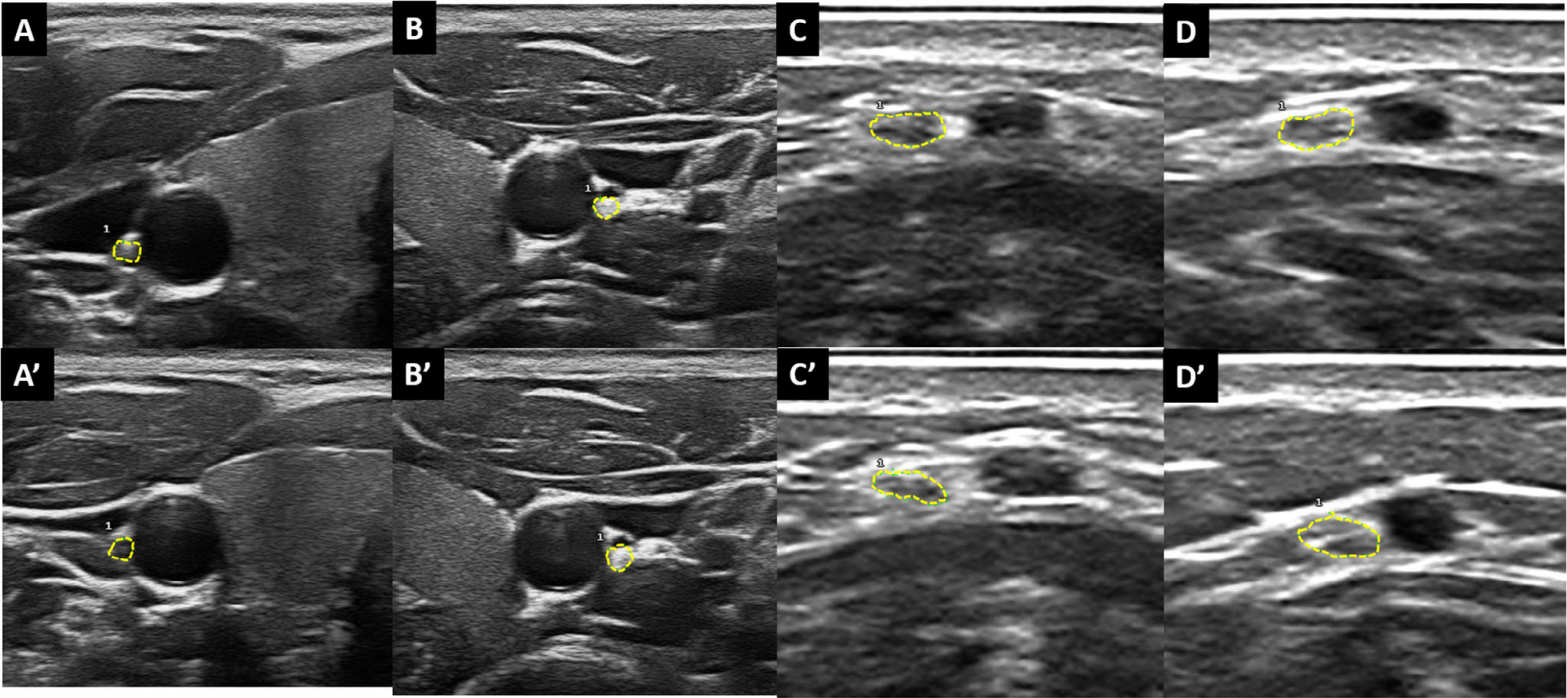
Ultrasound scans of nerves in a healthy subject, assessed at baseline (A, B, C, and D) and at the 24‐month follow‐up (A’, B’, C’, and D’). Panels A and A’ depict the right vagus nerve, B and B’ the left vagus nerve, C and C’ the right sural nerve, and D and D’ the left sural nerve. All measured cross‐sectional area (CSA) values are 2 squared millimeters. The CSA regions are delineated with fine yellow dashed lines.
